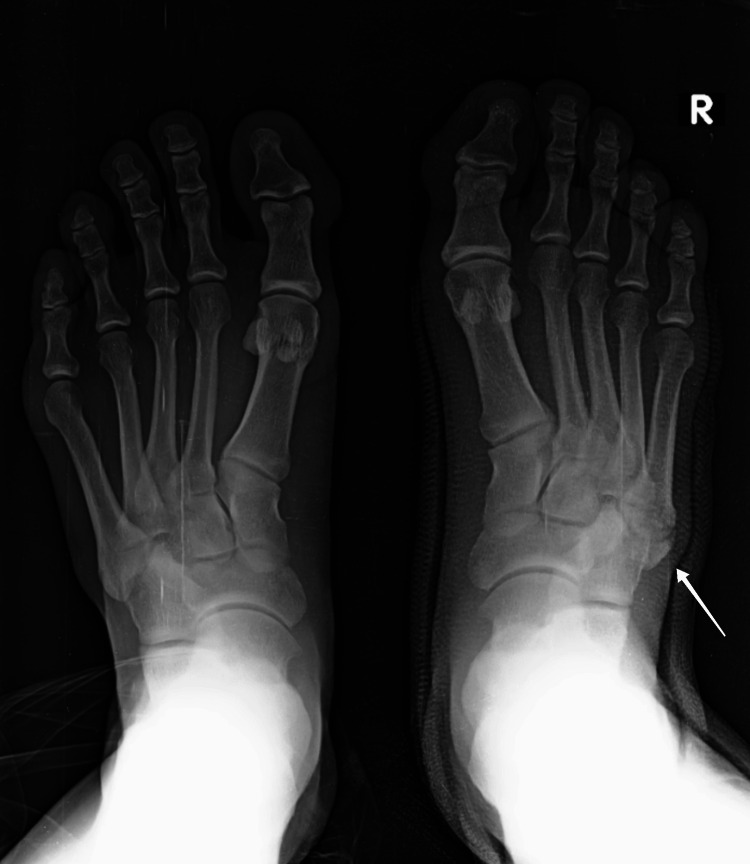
Bilateral foot anterior view radiograph. The white arrow points to OVP present in the base of the fifth metatarsal of the right foot only OVP: os vesalianum pedis
